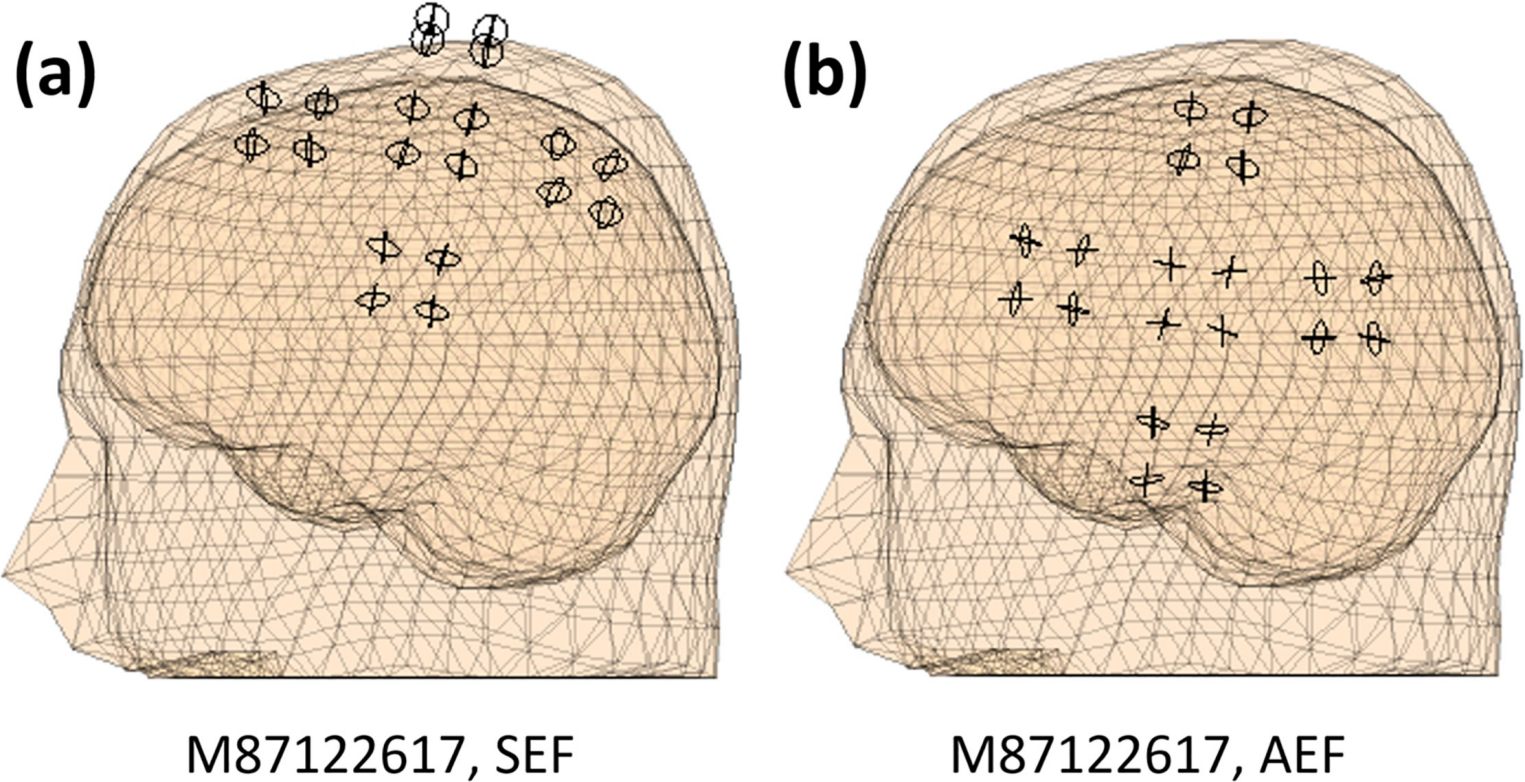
MEG-MRI co-registration. (a) SEF and (b) AEF experiments. The circles pertain to the location of OPM channels. For each MEG experiment the OPM array covers the cortex of interest.
